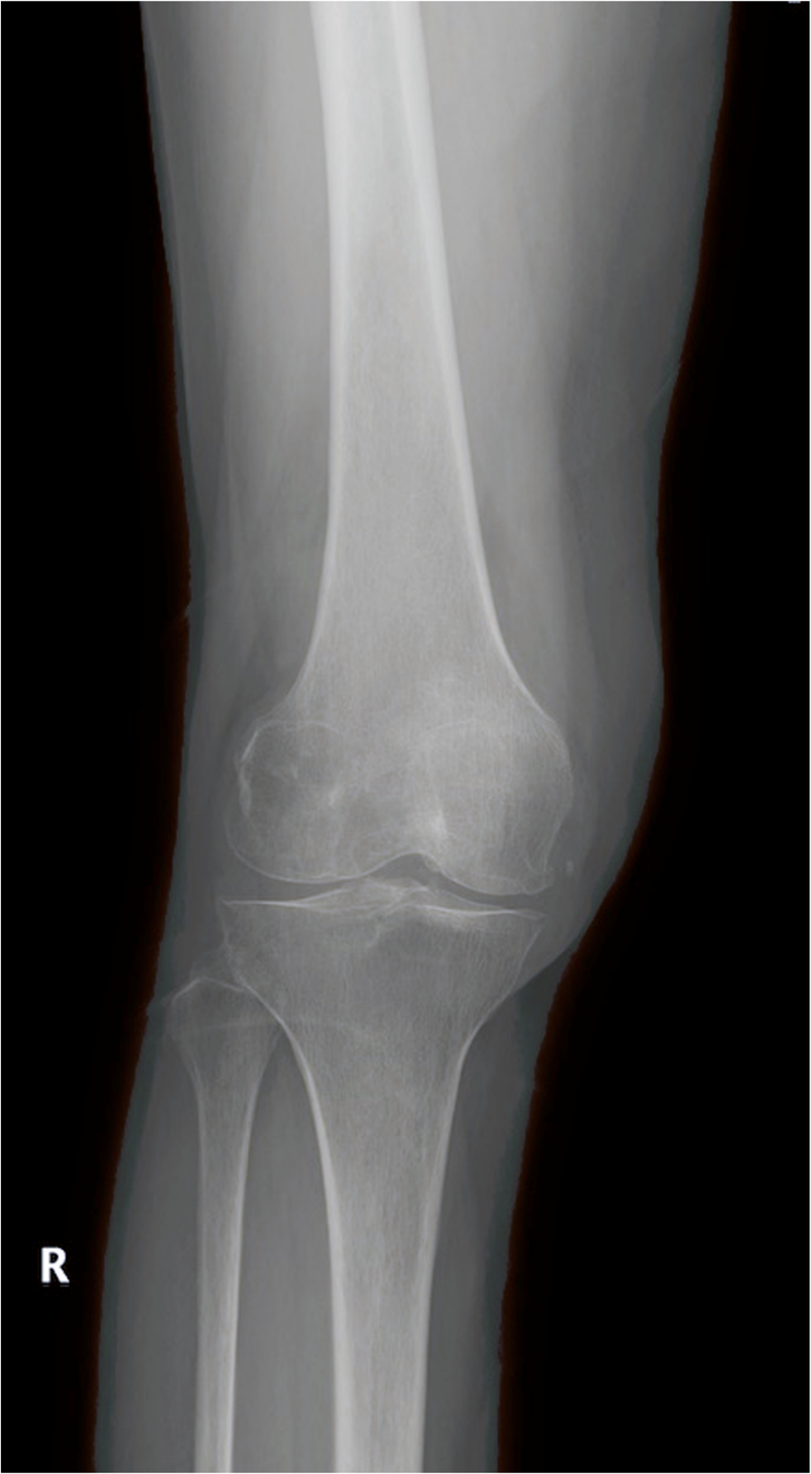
Typical X-ray findings of PVNS in the knee
